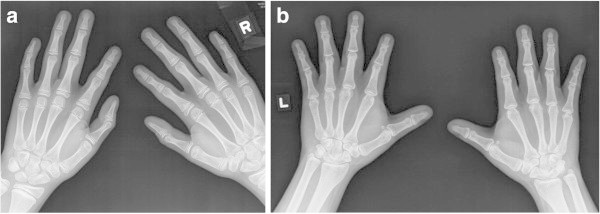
Bilateral hand X-ray of a 13 year old male (a) and a 22 year old male (b) with Ritscher-Schinzel Syndrome. a The fifth distal phalanges are hypoplastic, the bones are overtubulated (especially metacarpals 2-4) and the distal phalanges have prominent tufts. b Metacarpals 2-4 are overtubulated, the distal phalanges short, and the fifth distal phalanges are gracile.
